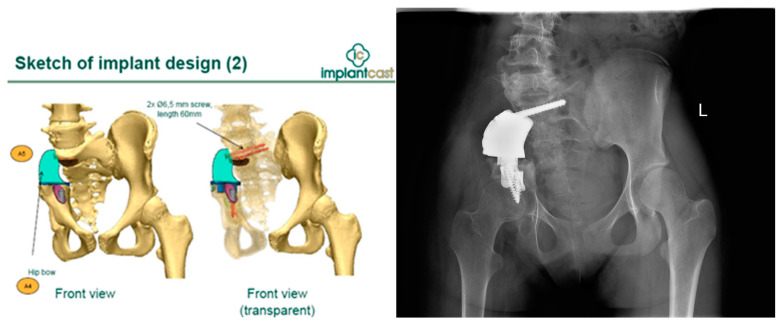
MUTARS 3D-printed implant design and postoperative X-ray view.
